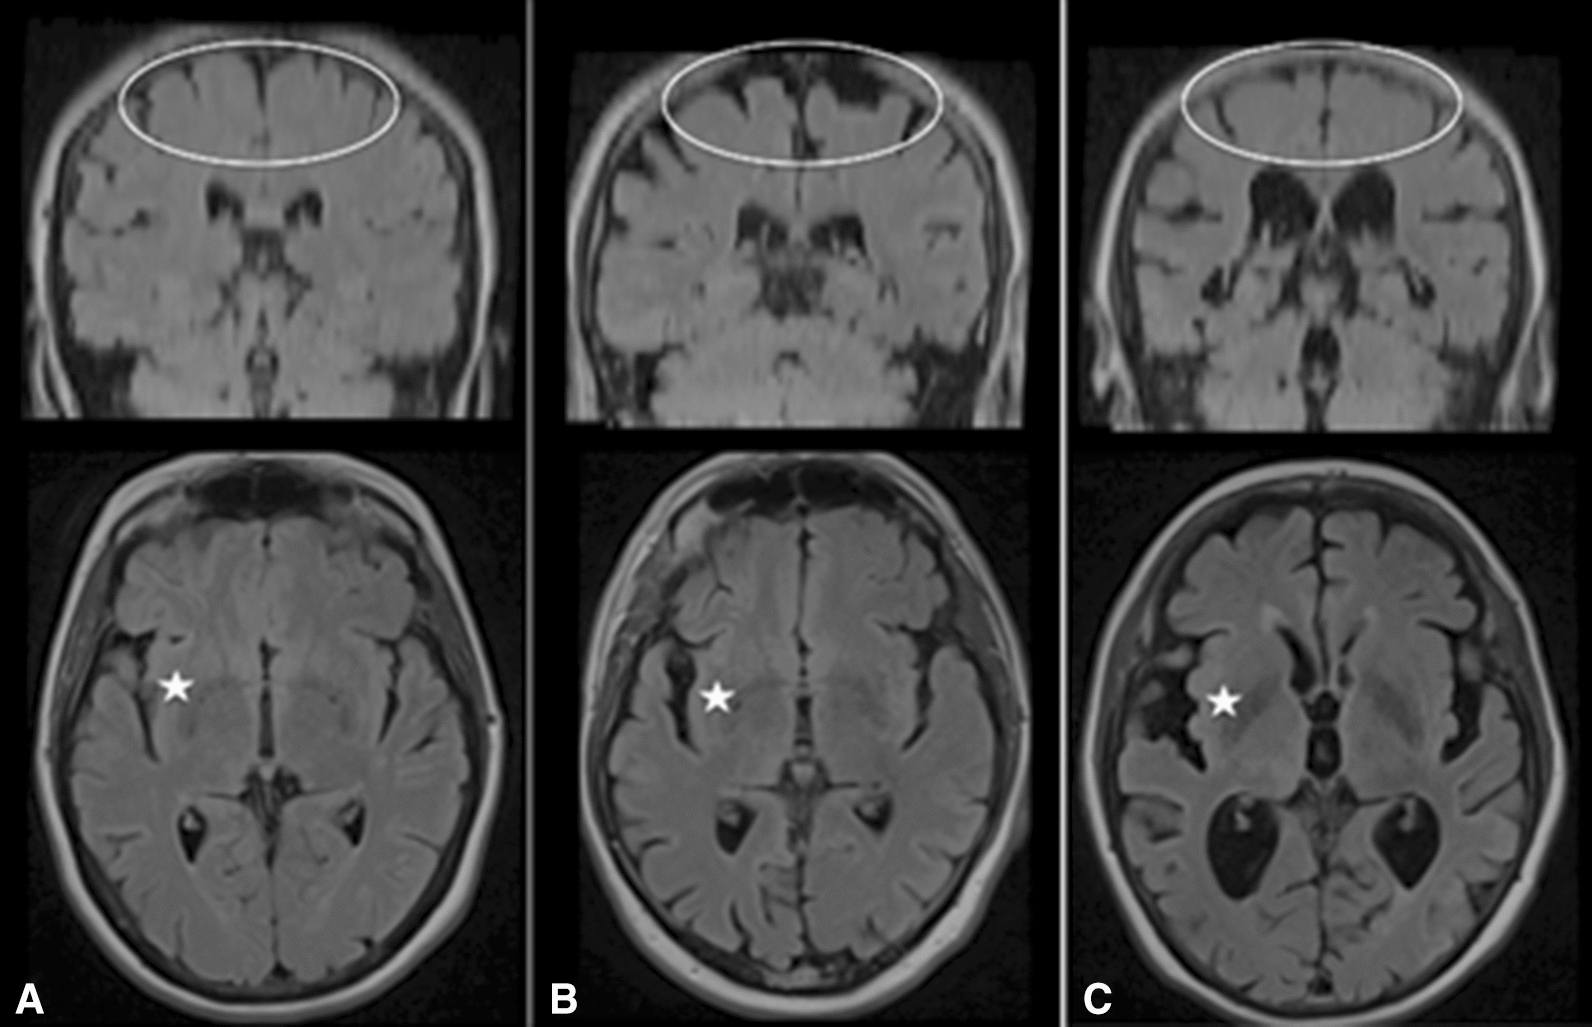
Examples of “normal,” unspecific “atrophy,” and “iNPH” subjects. T2-weighted Fluid inversion recovery (FLAIR) coronal (upper row) and axial (lower row) images of A “normal,” B unspecific “atrophy” (note atrophy of both the apex, Sylvian fissures, and mild enlargement of the lateral ventricles) and C “iNPH” (note the discrepancy between the relatively tight apex, wide Sylvian fissures and ventricles). *Ellipse* shows apex. One of the Sylvian fissures is located left of the *star*
